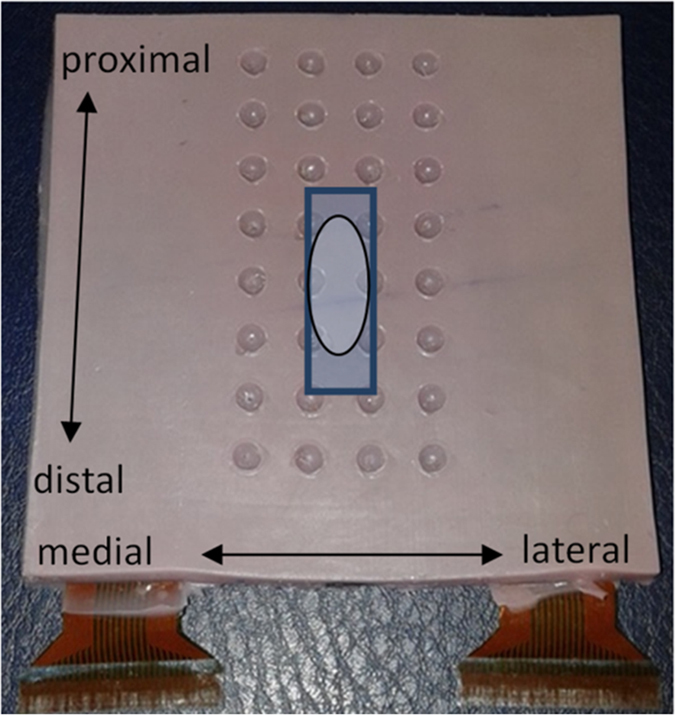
Multi-channel electrode transparent to ultrasound. The rectangle indicates the position of the ultrasound transducer whereas the oval indicates the electrode region which was compared as “at ultrasound beam”.
